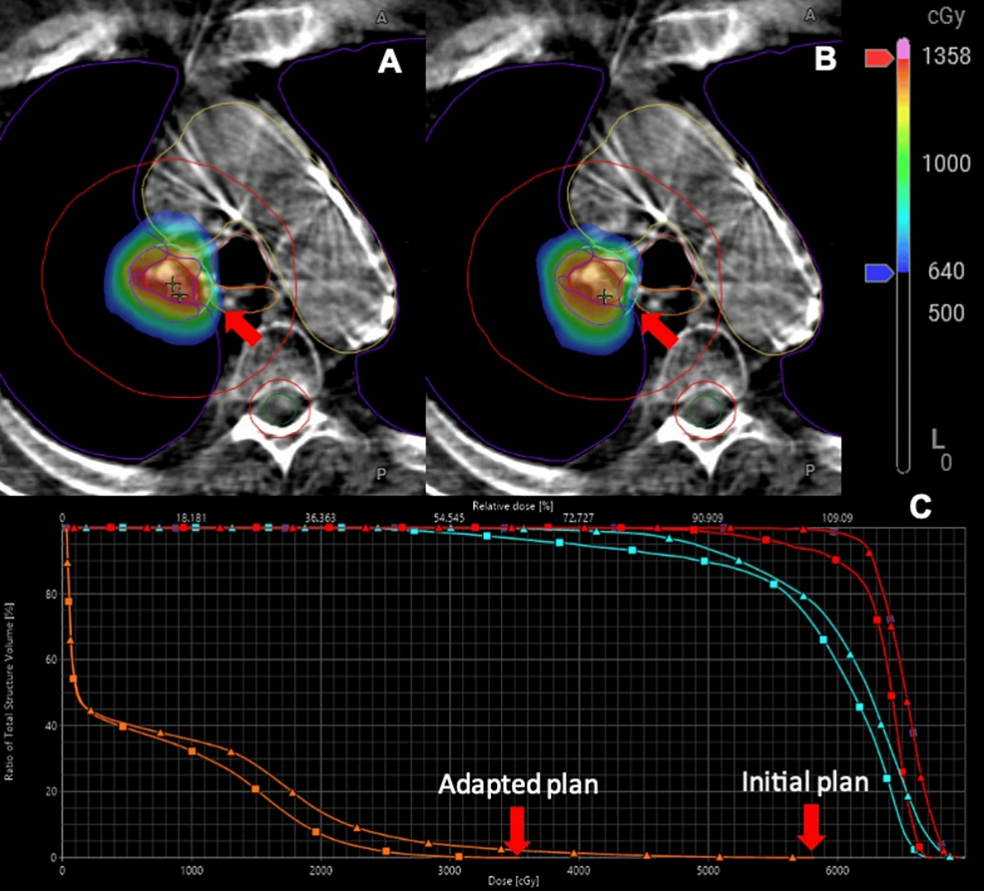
Initial and adapted plans Comparison of PI and PA using cone beam computed tomography (CBCT)-guided stereotactic adaptive radiotherapy (CT-STAR) for this patient with early-stage ultra-central non-small cell lung cancer (NSCLC). PI (A) and PA (B) for fraction three of treatment. In the PI, the esophageal constraint (V32Gy < 0.5cc) would have been violated, which was resolved using the PA. The dose to the esophagus, gross tumor volume (GTV) (red), and planning target volume (PTV) (cyan) are represented in the dose-volume histogram (DVH) (C) for both the PI (triangles) and PA (squares). Note that the dose on the CBCT images reflects the per-fraction dose, and the dose on the DVH represents the projected dose across all five fractions.
